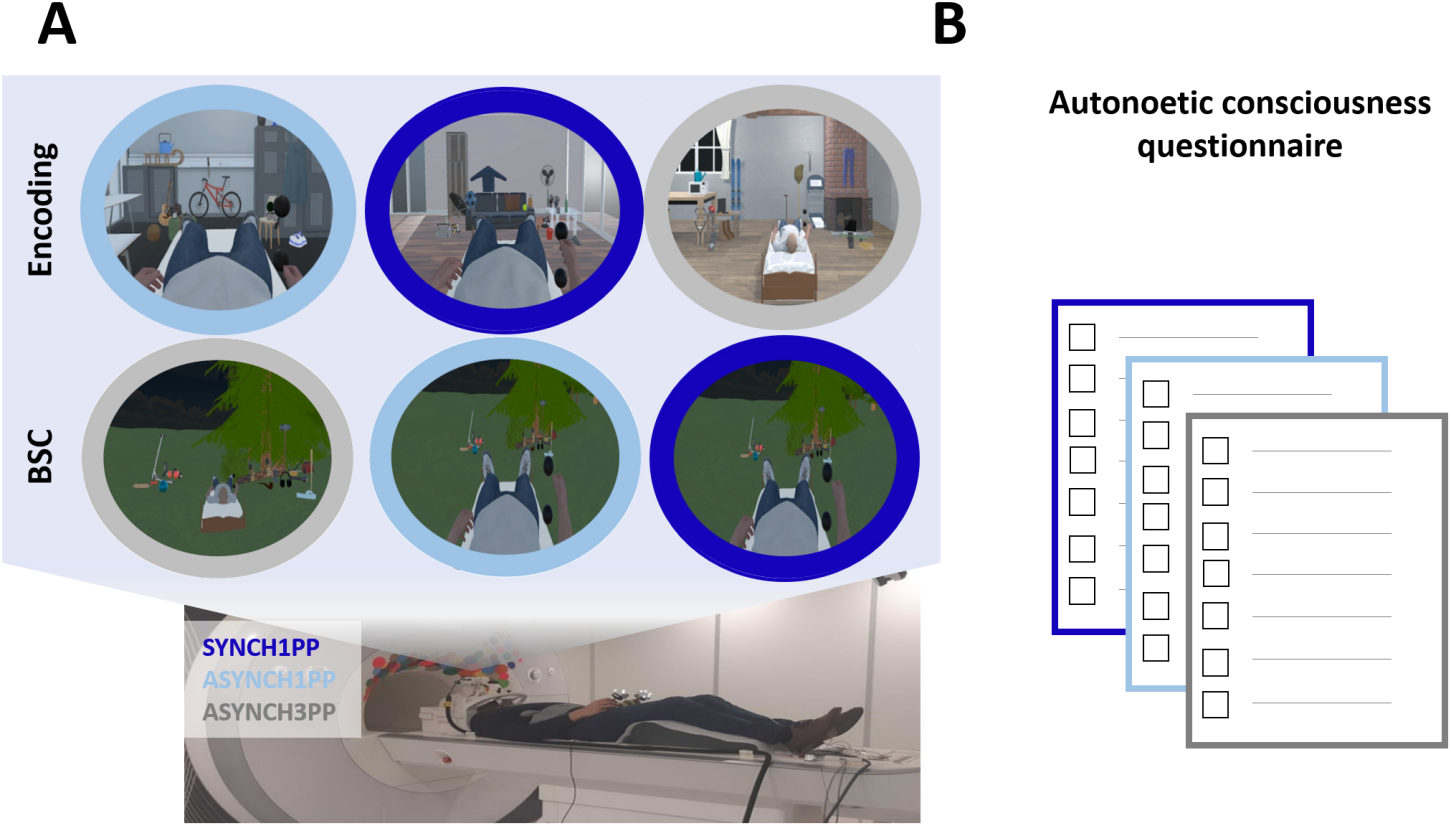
Experimental design. (A) Encoding and BSC assessment. Participants were in an MR scanner (main MRI study) or a Mock scanner (for the behavioral study) while observing different scenes in virtual reality. During encoding, participants moved their right arm while observing an avatar performing the same movement. Participant encoded one scene under synchronous first-person perspective (SYNCH1PP, preserved BSC, dark blue), one scene under asynchronous first-person perspective (ASYNCH1PP, light BSC manipulation, light blue), and one scene under asynchronous third-person perspective (ASYNCH3PP, strong BSC manipulation, grey). BSC was assessed immediately after encoding in a different fourth scene, where participants rated their sense of agency (SoA), body ownership (SoO), and control questions, for each condition. (B) Autonoetic consciousness assessment. One week later, we evaluated autonoetic consciousness (ANC) of participants using a series of questions derived from standardized questionnaires (“memory characteristic questionnaire”; MCQ, Johnson et al., 1988) and the “episodic autobiographical memory interview”; EAMI part B, Irish et al., 2008, 2011). See Table 1 for the detail of each item. The figure is adapted from Meyer, Gauthier, Potheegadoo, et al. (2024).
